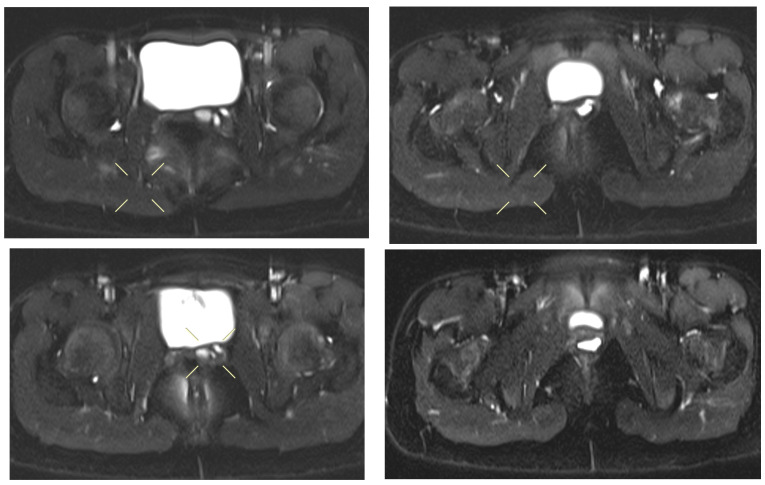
MRI pelvis in Case 4 showing uterine didelphys with an upper vaginal cystic structure communicating with the left uterine horn, resulting in narrowing of the upper vaginal cavity.
